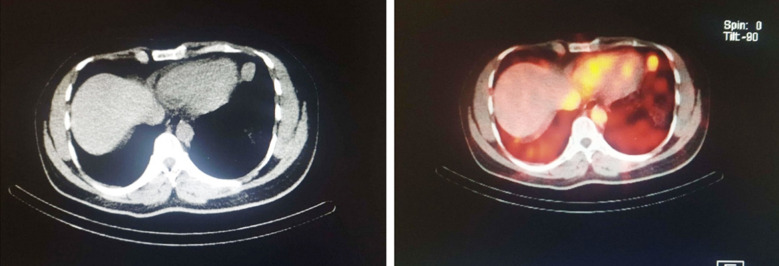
HRCT and 99m-Tc DRBC scan showing splenic nodule measuring 2.1x1.5cm in left mediastinum adjacent to cardiac apex.
